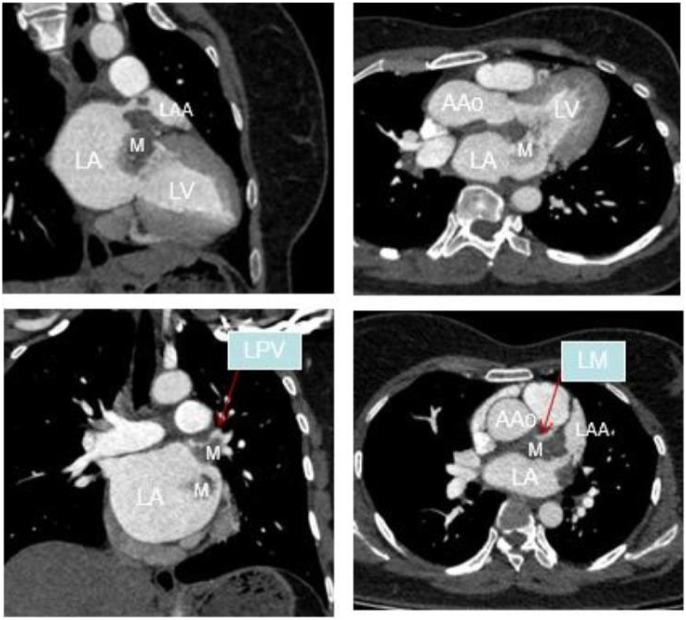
Computed tomography of arterial intimal sarcoma.
